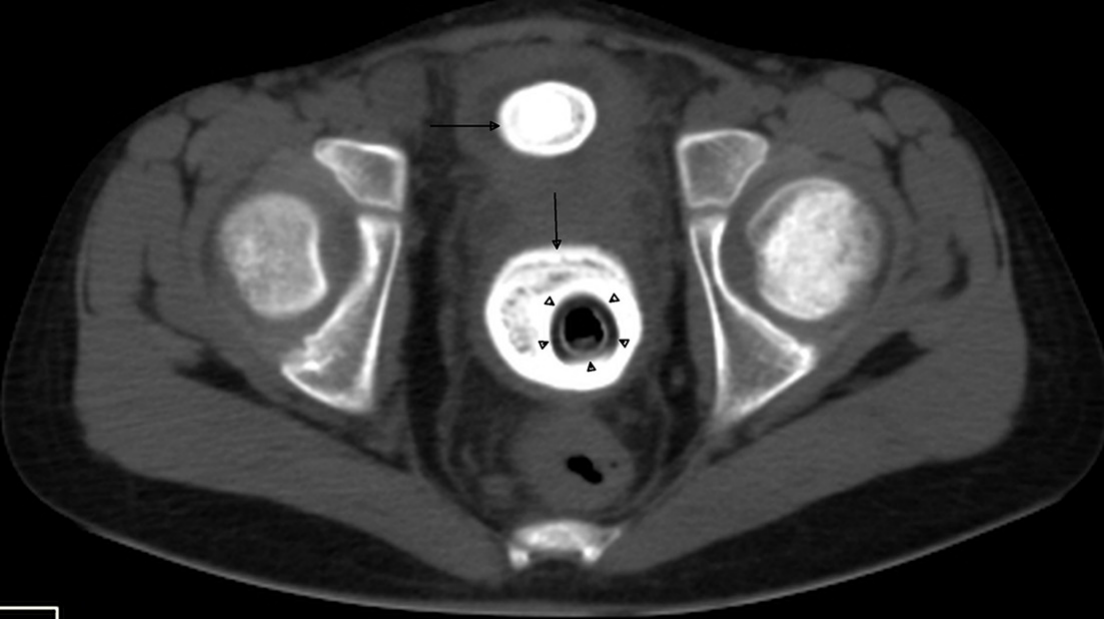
Axial non-contrast CT scan of the pelvis showing a vesical calculus (horizontal arrow) and a vaginolith (vertical arrow) surrounding the vaginal foreign body (arrowheads).
